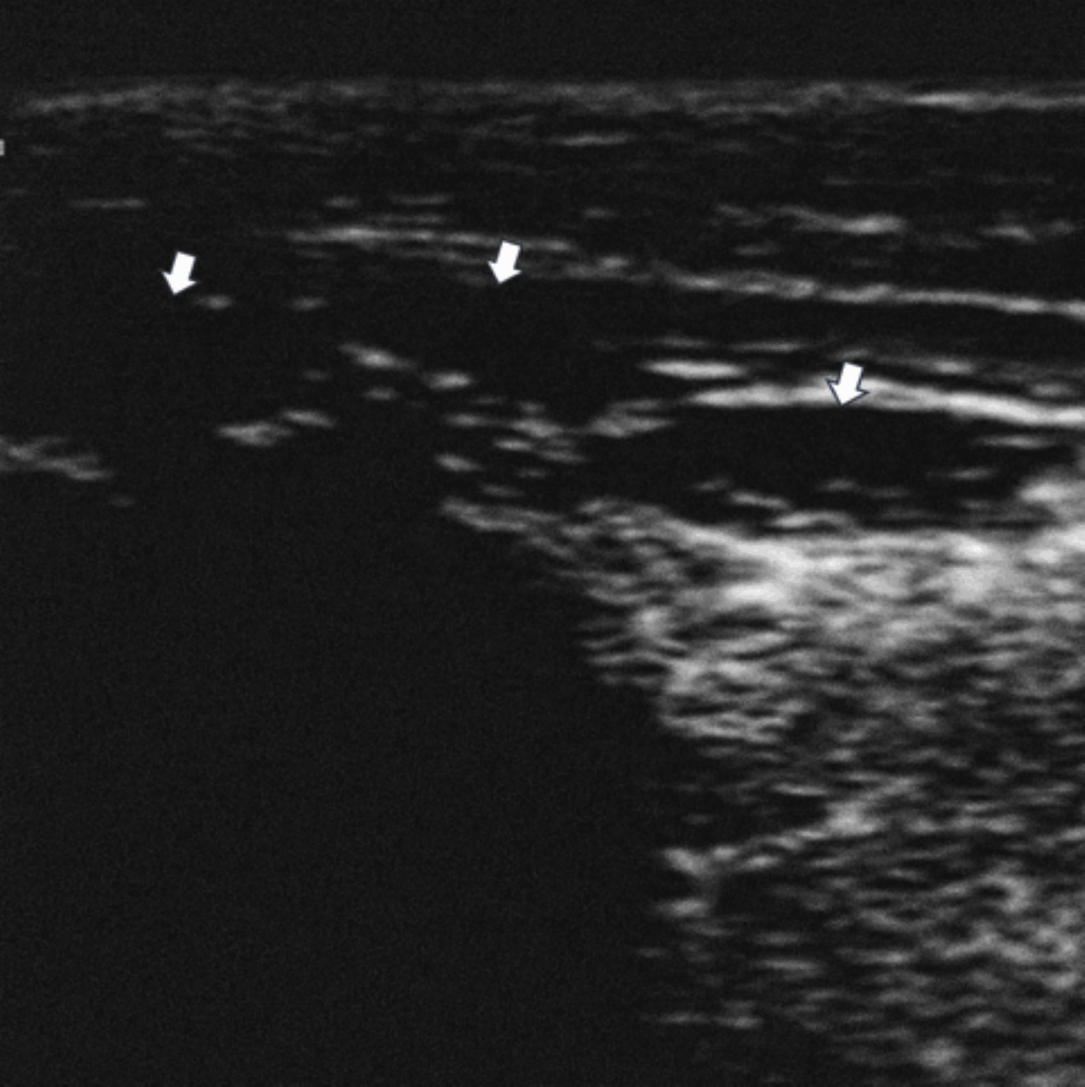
Sonogram of the midfoot showing three differently sized well-defined fusiform hypoechoic nodules (arrows) arising within plantar fascia
